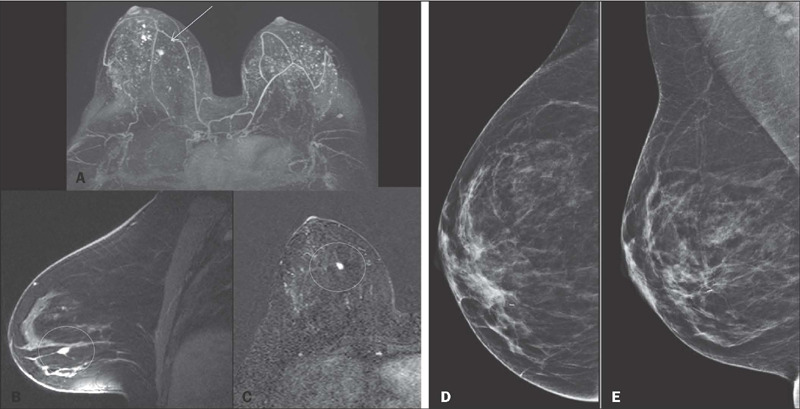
A 42-year-old patient who underwent mammography and MRI for breast
